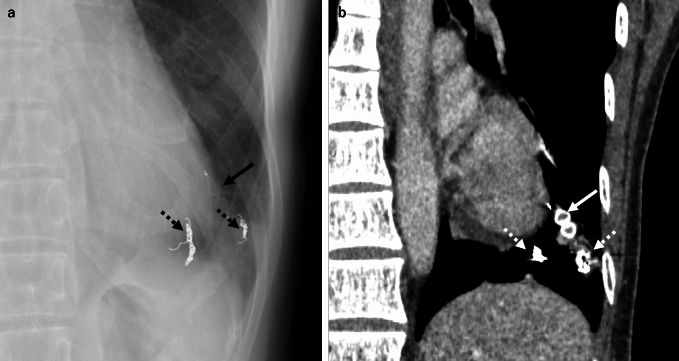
Vascular plug and coil placed in the pulmonary artery to treat a pulmonary arteriovenous fistula. A woman in her 40 s underwent the embolization of a pulmonary arteriovenous fistula in the left lower lobe of her lung with the use of a vascular plug and coils. Chest radiography and chest CT confirmed the presence of the vascular plug (a, b, arrow) and coils within the left pulmonary artery (a, b, dot arrow)
